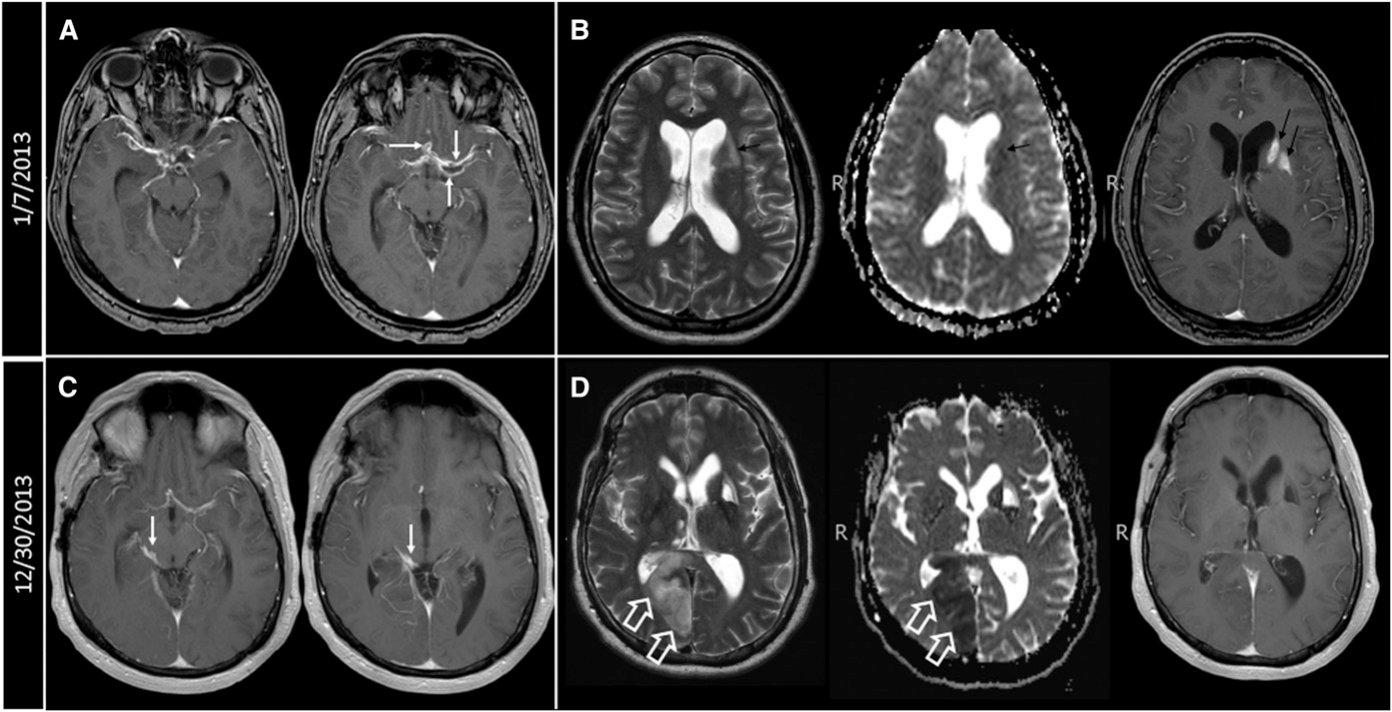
(A) Postcontrast T1-weighted images showing abnormal enhancement along the courses of the MCAs and ACAs bilaterally with associated cystic changes (white arrows). (B) Axial T2, axial apparent diffusion coefficient (ADC) maps, and axial postcontrast T1-weighted images showing subacute infarcts in the left basal ganglia and centrum semiovale (black arrows). (C) Abnormal enhancement in the right side of the perimesencephalic cistern (white arrows) along the course of the right posterior cerebral artery (PCA). (D) Axial T2, axial ADC maps, and axial postcontrast T1-weighted images showing acute infarction in the right medial occipito-parietal region (white hollow arrows).
